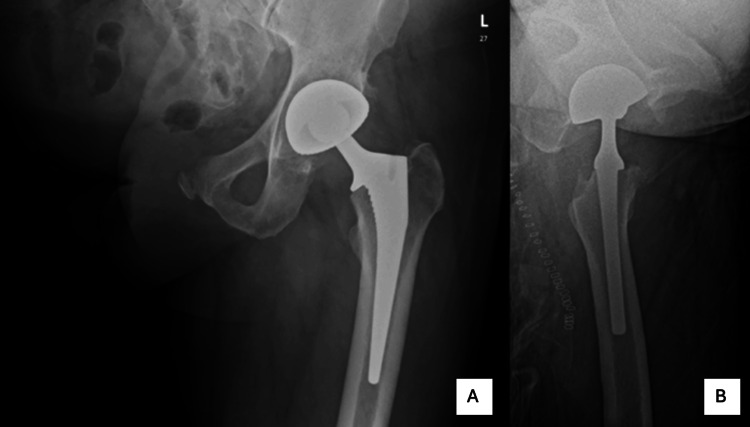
Postoperative X-ray of the left hip. A: Lateral X-ray of the left hip after total hip replacement. B: Anterior X-ray of the left hip after total hip replacement.
